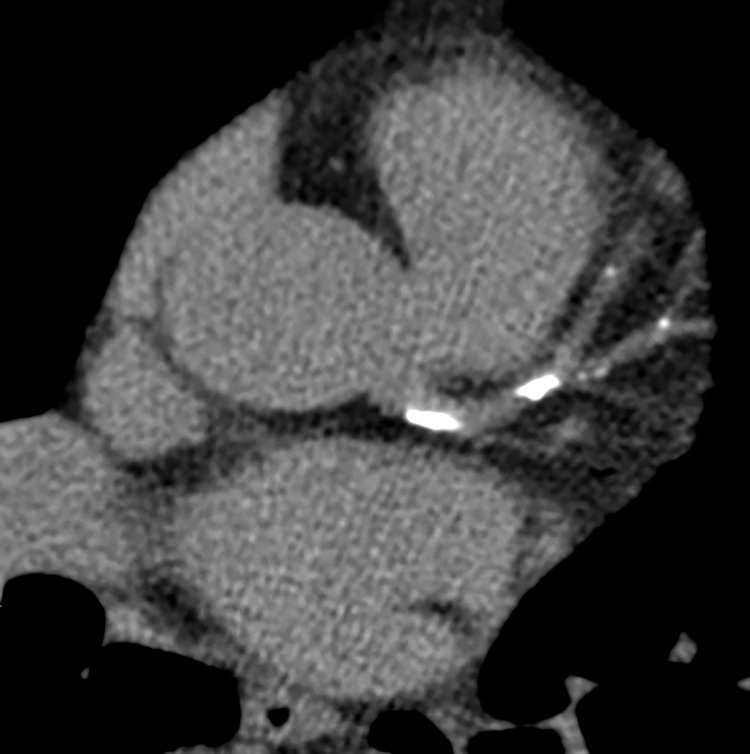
Coronary calcium scoring in a 64-year-old woman with diabetes mellitus and intermittent chest pressure. Selected axial CT image from a coronary calcium score examination shows calcification within the left main coronary artery, left anterior descending (LAD) coronary artery, and diagonal branches. The total quantified coronary calcium was severe, yielding an Agatston score of 622, which represents the 95th percentile for patients of the same age, gender, and race/ethnicity who are free of clinical cardiovascular disease and treated diabetes per the MESA coronary calcium calculator *(https://www.mesa-nhlbi.org/Calcium/input.aspx).*
